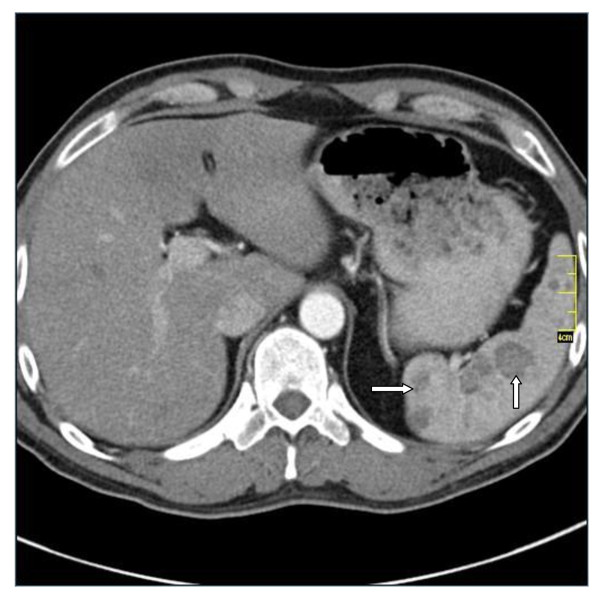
Computed tomography showing multiple hypoattenuating lesions (arrows).
